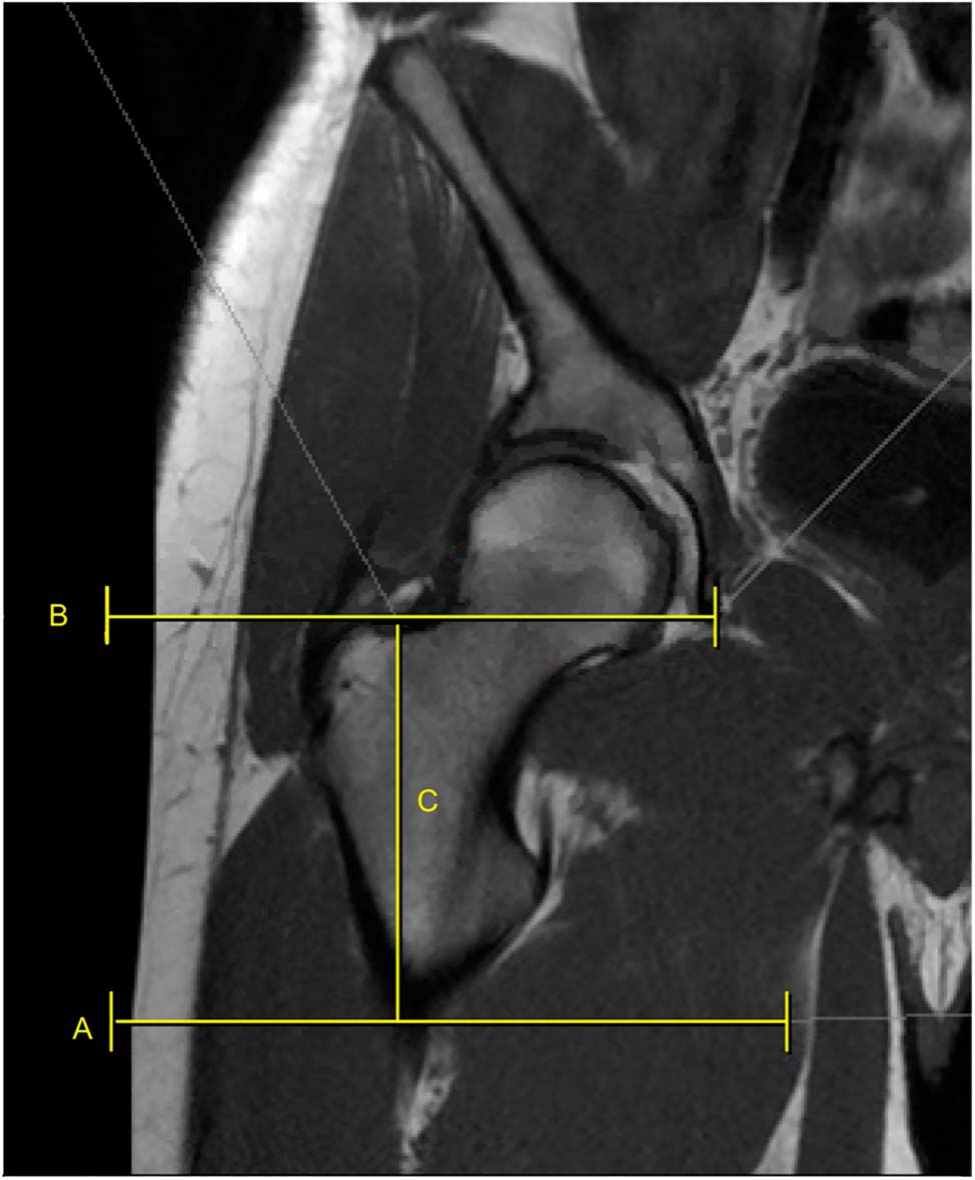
Radiograph showing line A—proximal aspect of GS, line B—tip of GT, and line C—distance between GS and tip of GT. GS = gluteal sling, GT = greater trochanter
